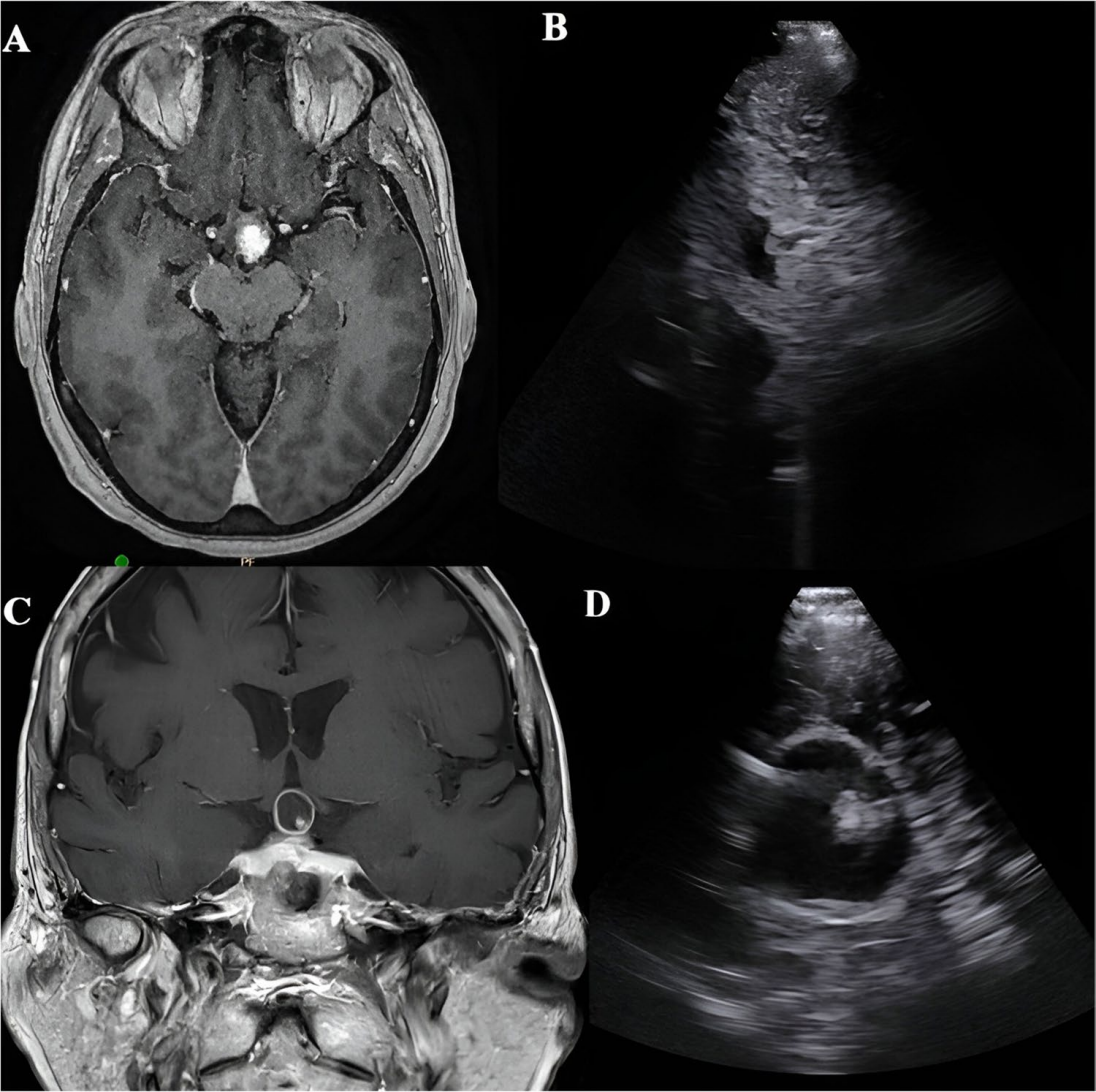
Preoperative MRI images of 2 other cases of PCP with corresponding intraoperative IOUS image (all taken before the dural incision): A. and B.- Preoperative axial T1 with contrast MRI image and the corresponding intraoperative IOUS image of a case of PCP (third patient in the series), C. and D.- Preoperative coronal T1 with contrast MRI image and the corresponding intraoperative IOUS image of another case of PCP (fourth patient in the series)
